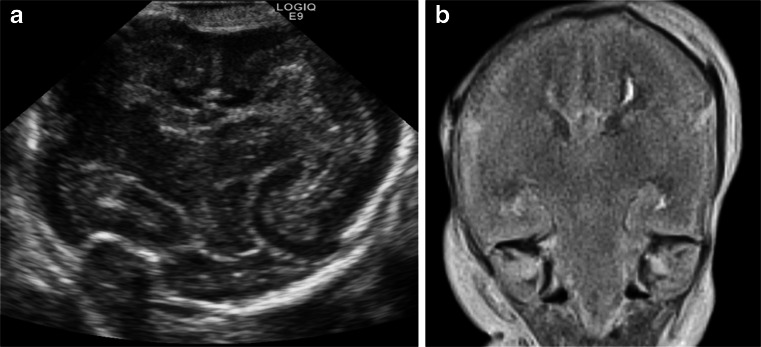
Postmortem US and MRI in a female foetus of 22 weeks gestation. a Cranial postmortem US. b Corresponding coronal T2-weighted postmortem MRI. Both show normal intracranial appearances. Note the minor deviation of the brainstem in the postmortem US image, caused by positioning
